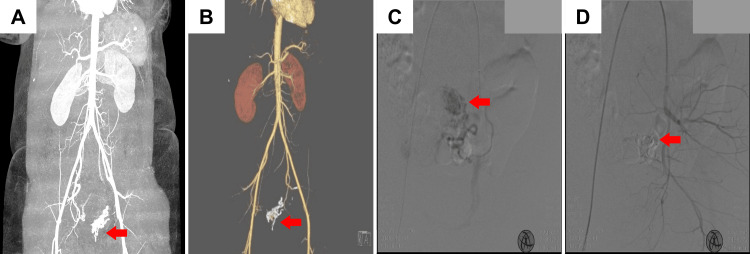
Computed tomography (CT) and angiogram of patient 1 (A, B) CT pelvis showing the UAVM (red arrow) involving the left lateral uterine wall and the endometrium. (C) Super-selective cannulation of the distal uterine artery was performed using the microcatheter. The angiogram shows the arteriovenous communications (red arrow) arising from the left uterine artery. (D) Post-uterine artery embolization shows complete occlusion (red arrow) of the UAVM and glue cast. UAVM: uterine arteriovenous malformation
